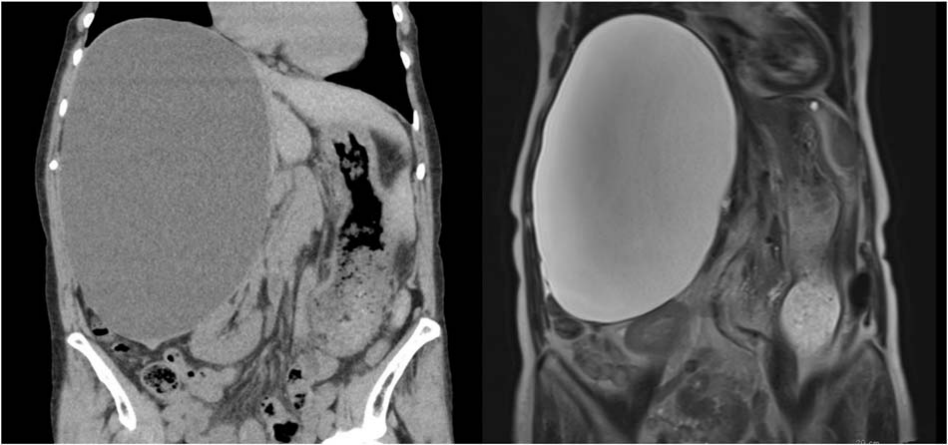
A 13 × 17 × 24 cm hepatic cyst in the right lobe is observed in both CT and MRI.
